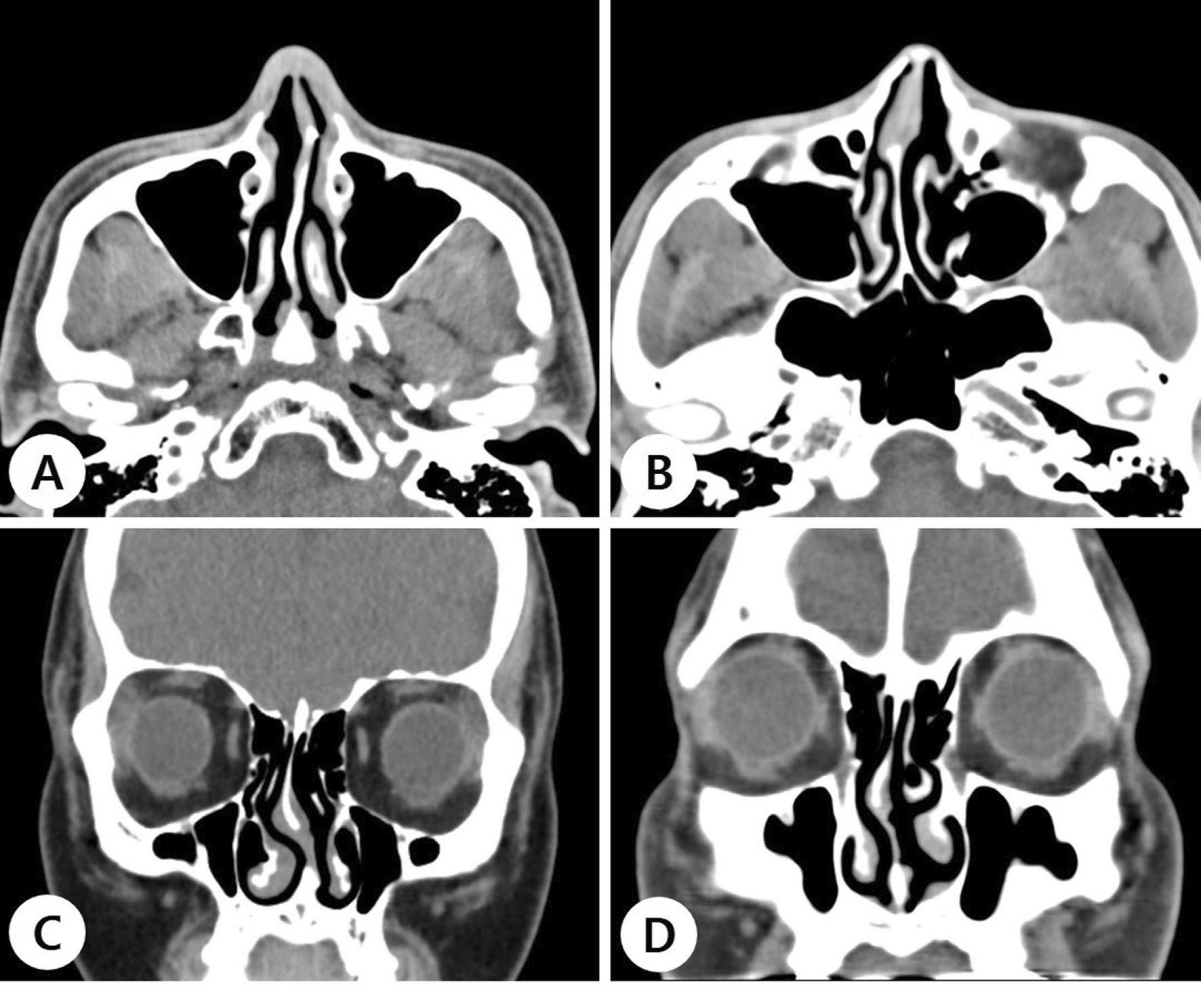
Types of nasal septal deviation. Axial (A, B) and coronal (C, D) computed tomography images reveal S- (A, C) and C-shaped (B, D) deviations in the anteroposterior and cephalocaudal directions, respectively.
